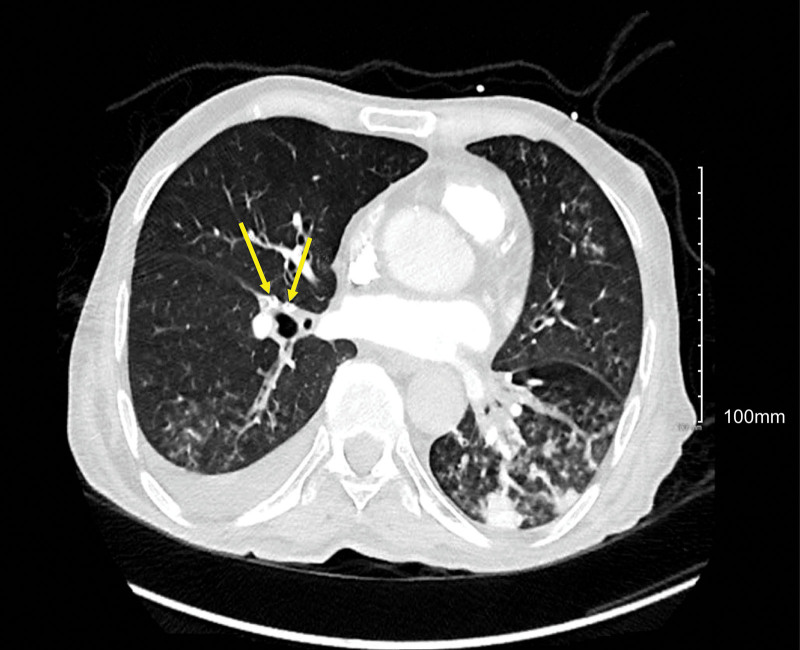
Chest CT scan showing air bubbles in the right lower lobe segmental pulmonary artery. CT = computed tomography.
